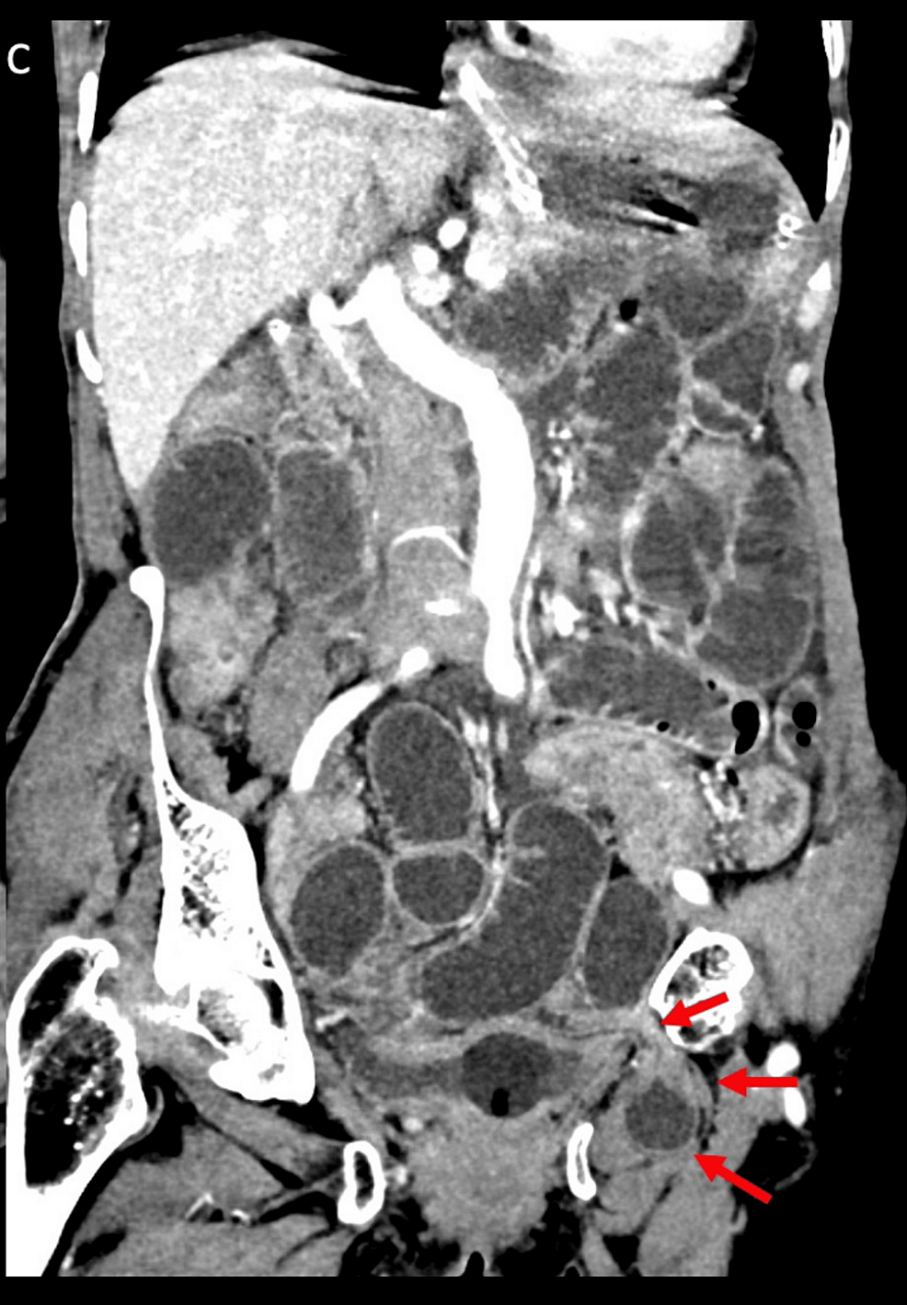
Contrast-enhanced CT showed obturator hernia in oblique coronal view Oblique coronal image showing the whole course of the obturator hernial sac (red arrows) along with proximally dilated jejunal and ileal loop.
